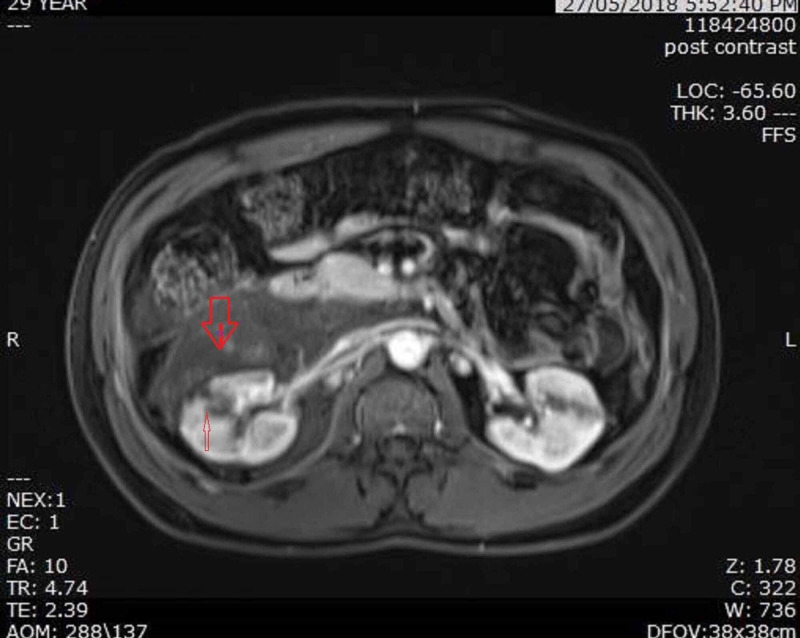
MRI showing large perinephric hematoma (arrow head) and a small hemorrhagic cyst ( arrow) in anterior renal cortex communicating with perinephric hematoma.
